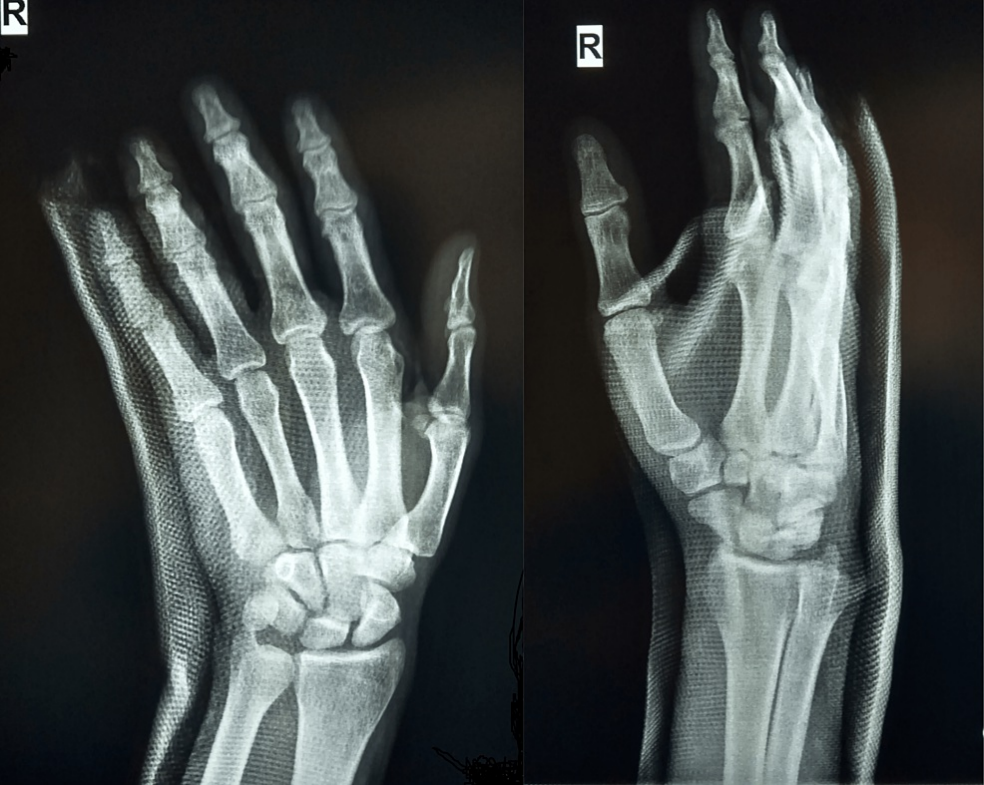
Radiograph (AP/lateral view) after the closed reduction and stabilization with ulnodorsal splint AP: Anteroposterior
